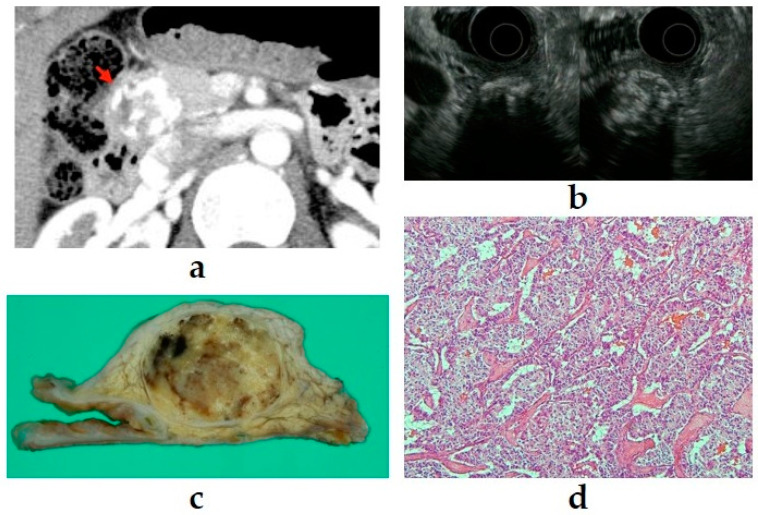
(a) Contrast-enhanced computed tomography (CT): a tumor (arrow) with calcification components is shown at the pancreatic head. (b) Endoscopic ultrasound (EUS): a well-defined mass with a heterogeneous appearance and peripheral rim calcification with posterior acoustic shadowing. (c,d) The imaging findings suggest a solid pseudopapillary neoplasm, but pathologically, it is a neuroendocrine tumor G1 (100×).
